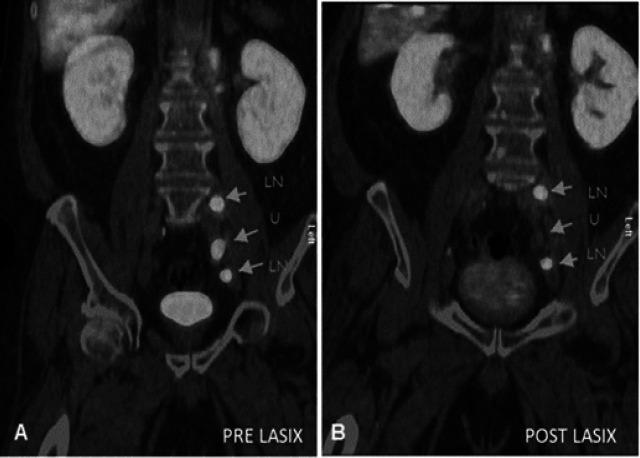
Coronal Fused PET/CT Images Showing Three Lesions in Athe Pre Lasix Study (Arrows), B) Post Lasix images show clearance of urine activity showing only two residual lesions. Urine uptake =U, Lymph node uptake=LN
